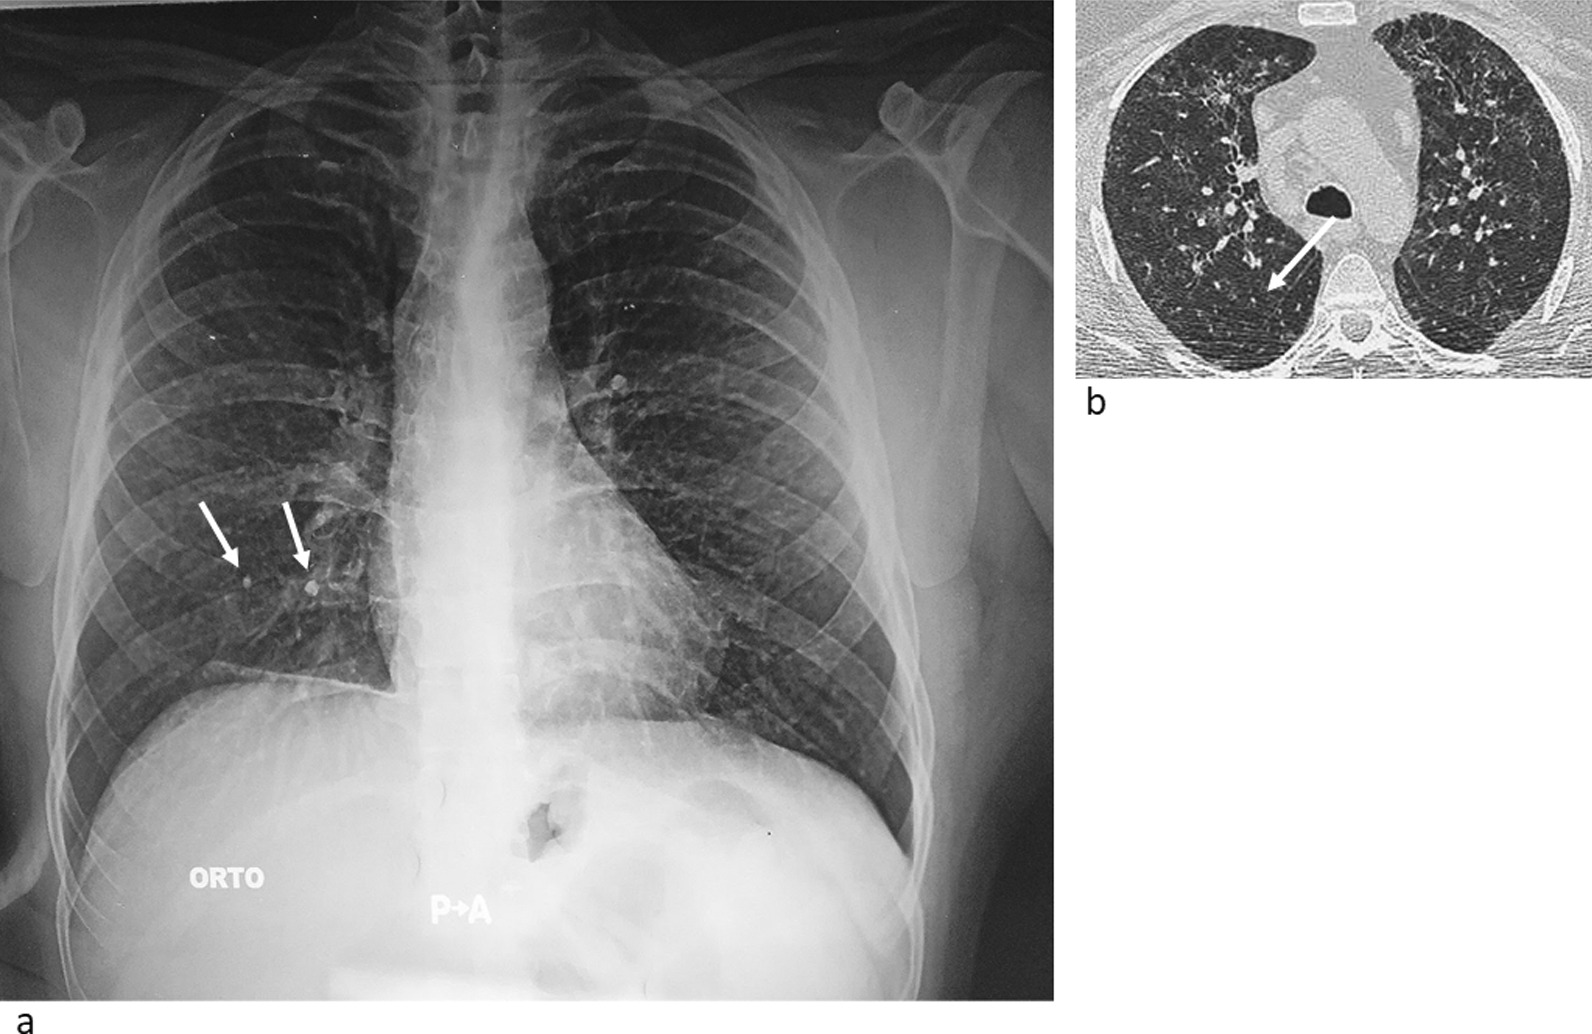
a Chest X-ray showing radiopacity in the mid-apical fields and fibrotic findings b Chest High Resolution Computerized Tomography (HRCT) showing miliary micronodulation, bilaterally
